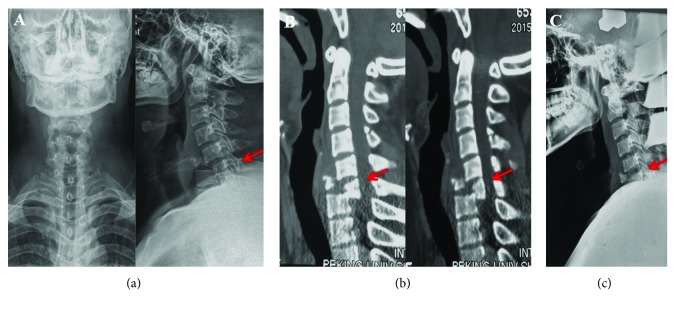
(a) Cervical spine X-ray. (b) Cervical spine CT. (c) X-ray under skull traction.
